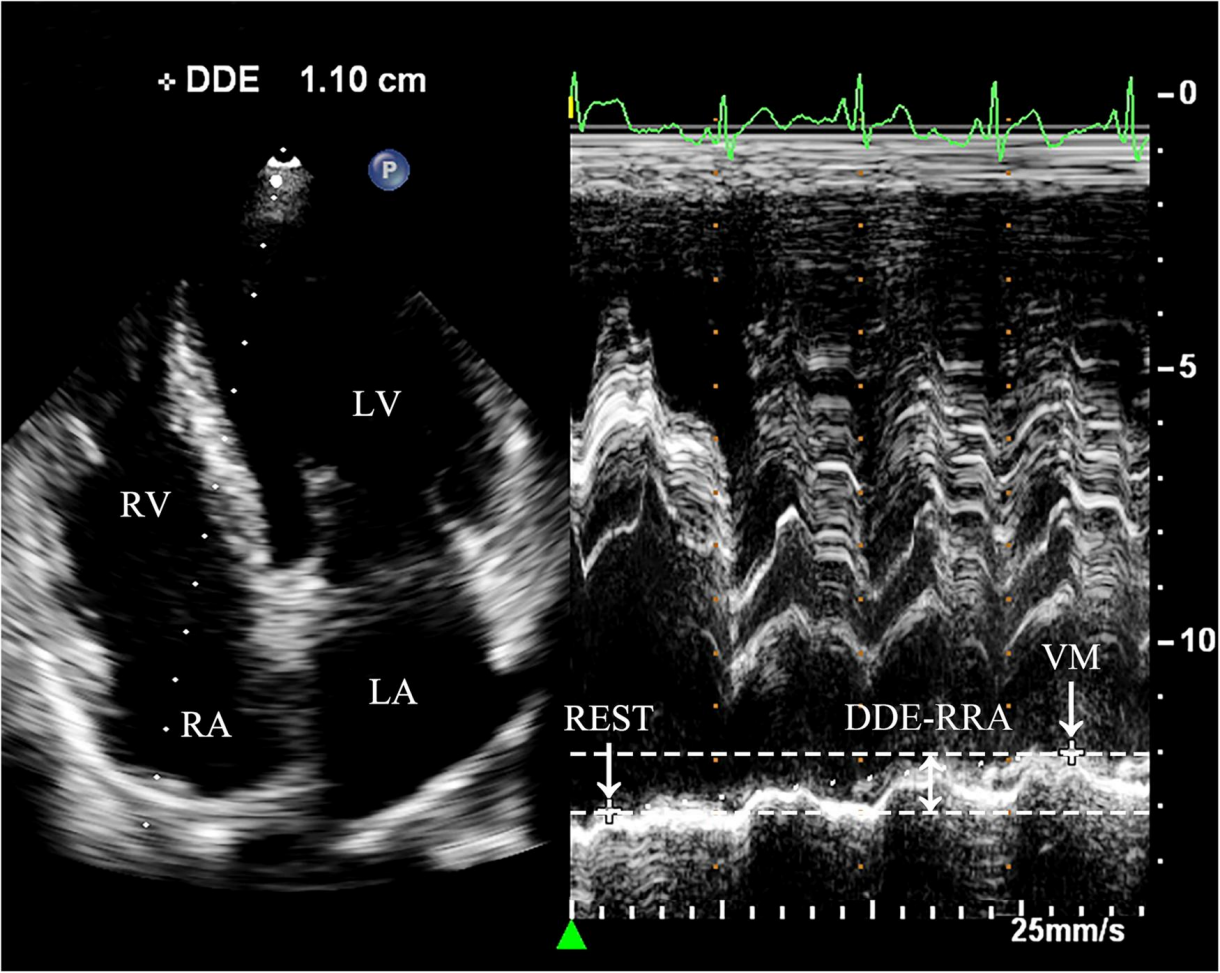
Measurement of DDE-RRA. Dramatic diaphragmatic downward excursion is observed. In the apical four-chamber view, the sampling line is placed across the top of the right atrium to obtain the M-mode motion curve of the diaphragm. REST refers to the moment right before the subject initiates the Valsalva maneuver, when they are in a baseline or resting state. VM refers to the point when the subject is holding their breath for 10 s during the Valsalva maneuver, just before releasing the breath. DDE-RRA (double-headed arrow) is the measured value representing the difference in the diaphragm's position between two distinct time points. REST, at rest; VM, Valsalva maneuver; LA, left atrium; LV, left ventricle; RA, right atrium; RV, right ventricle; DDE-RRA, diaphragmatic downward excursion at the roof of the right atrium.
